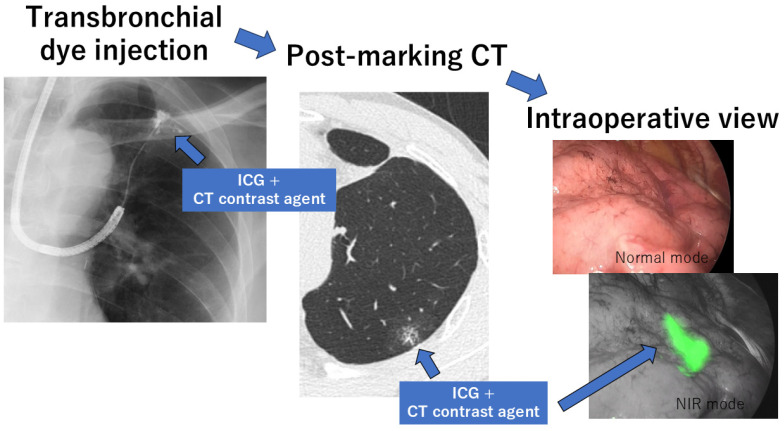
Indocyanine green virtual-assisted lung mapping (ICG-VAL-MAP). CT: computed tomography, NIR: near infrared.
